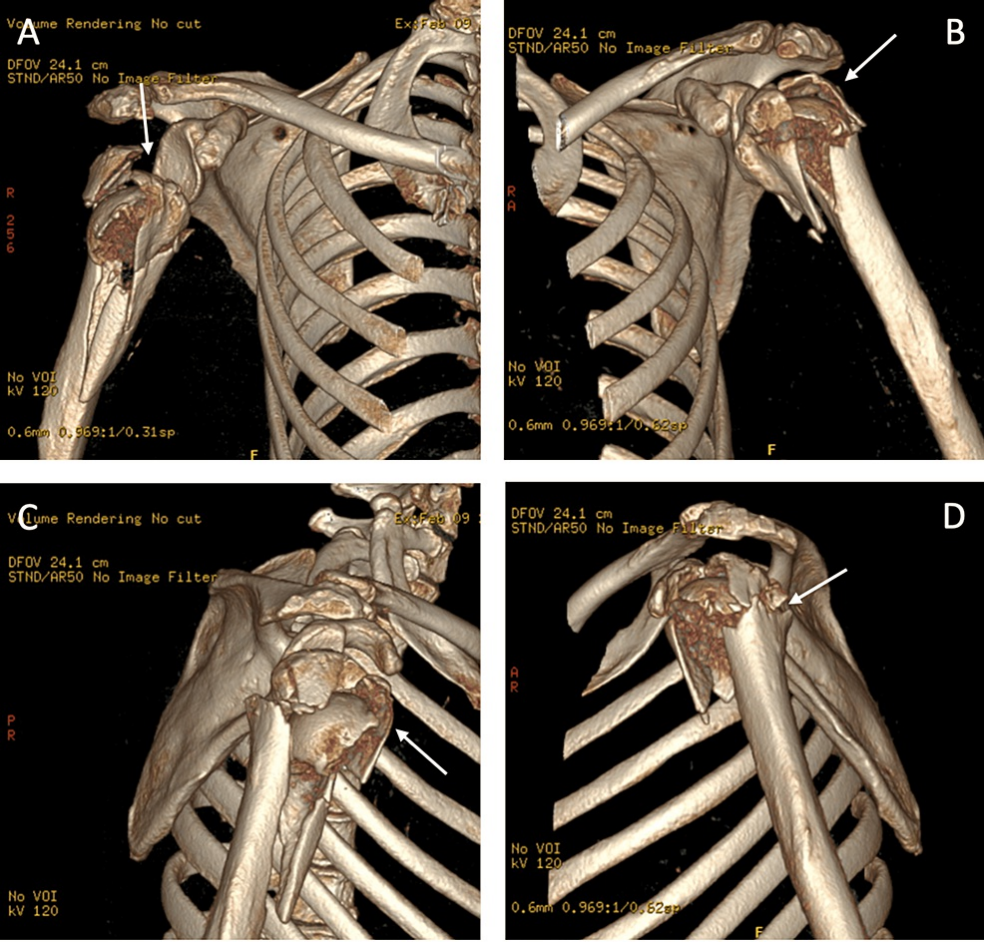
Pre-operative CT 3D reconstruction of the right (A, B) and left (C, D) shoulders demonstrating the comminution of fracture. White arrows point towards the fracture sites. CT: Computed tomography.
